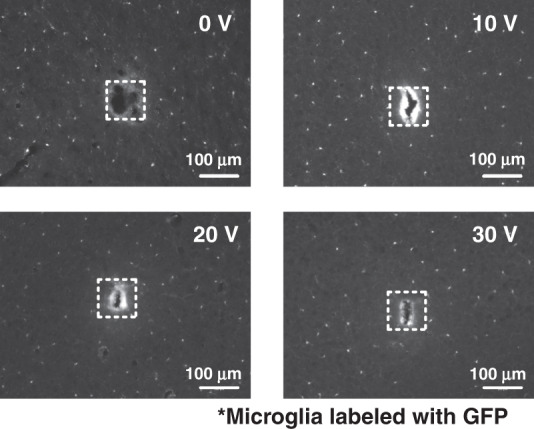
Fluorescence images of brain sections cut parallel to the cortical surface showing insertion sites ~1 h after inserting and removing the probe with different ultrasonic driving voltages. The white dotted square represents the probe tip size. The visible cells are microglia labeled with GFP
